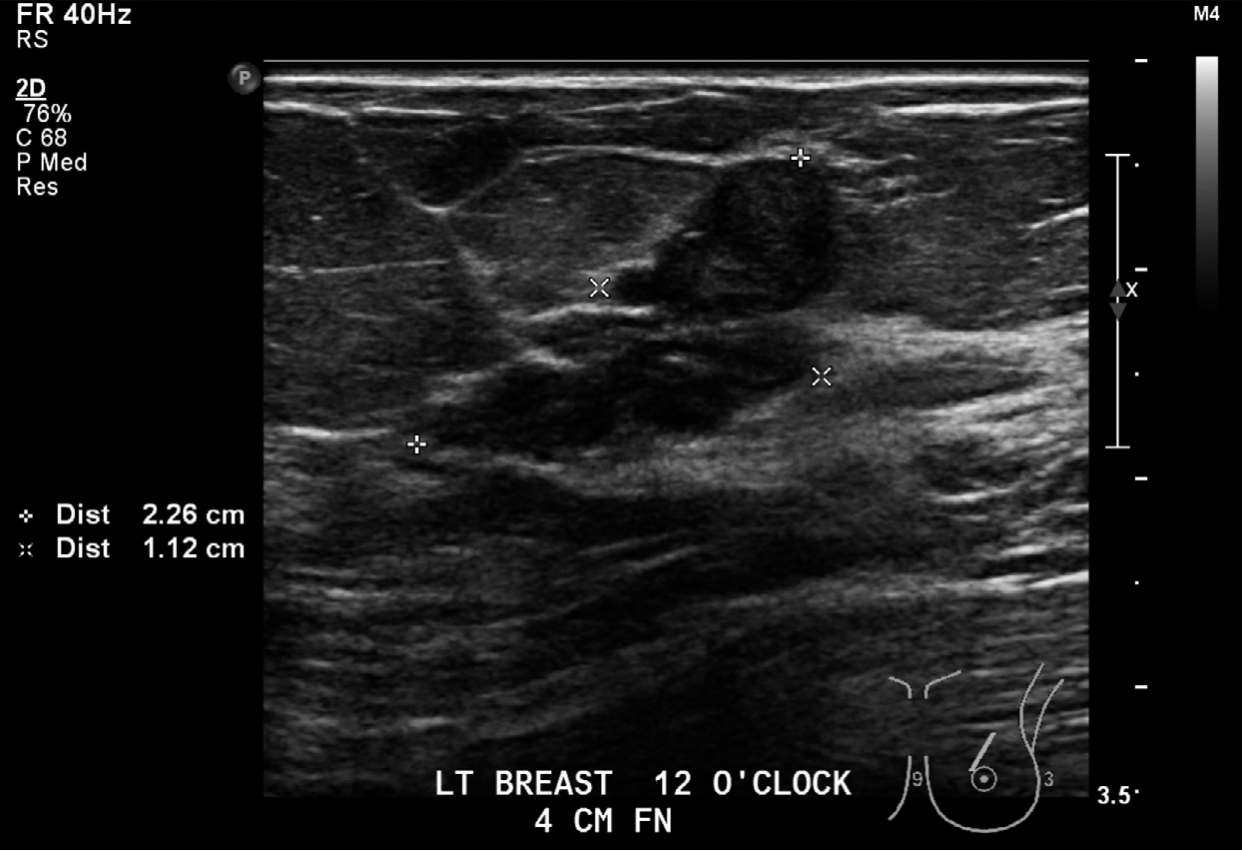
Ultrasound image demonstrating a known papillary lesion. Image courtesy of BreastScreen ACT.
